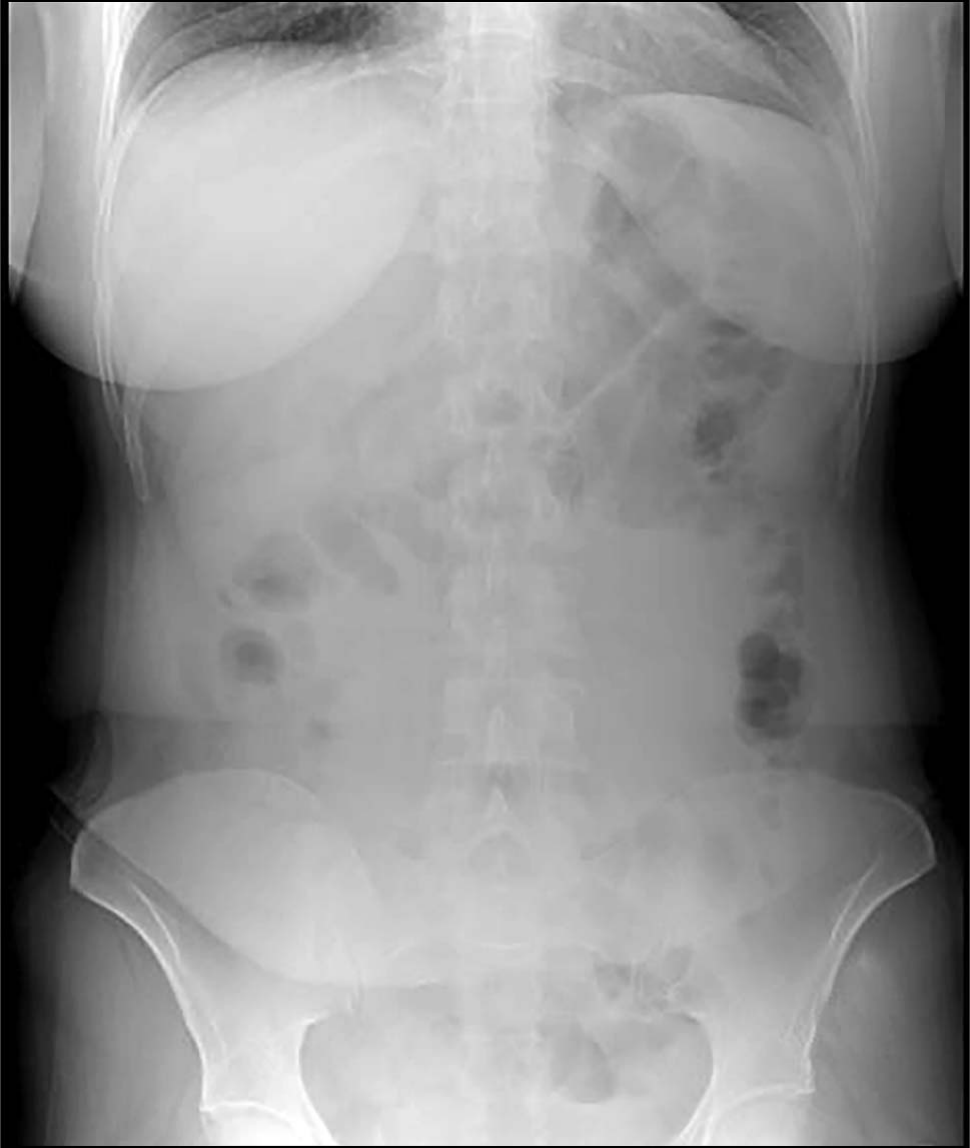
X-ray abdomen erect showing no signs of pneumoperitoneum after endoscopic removal of gossypiboma.
